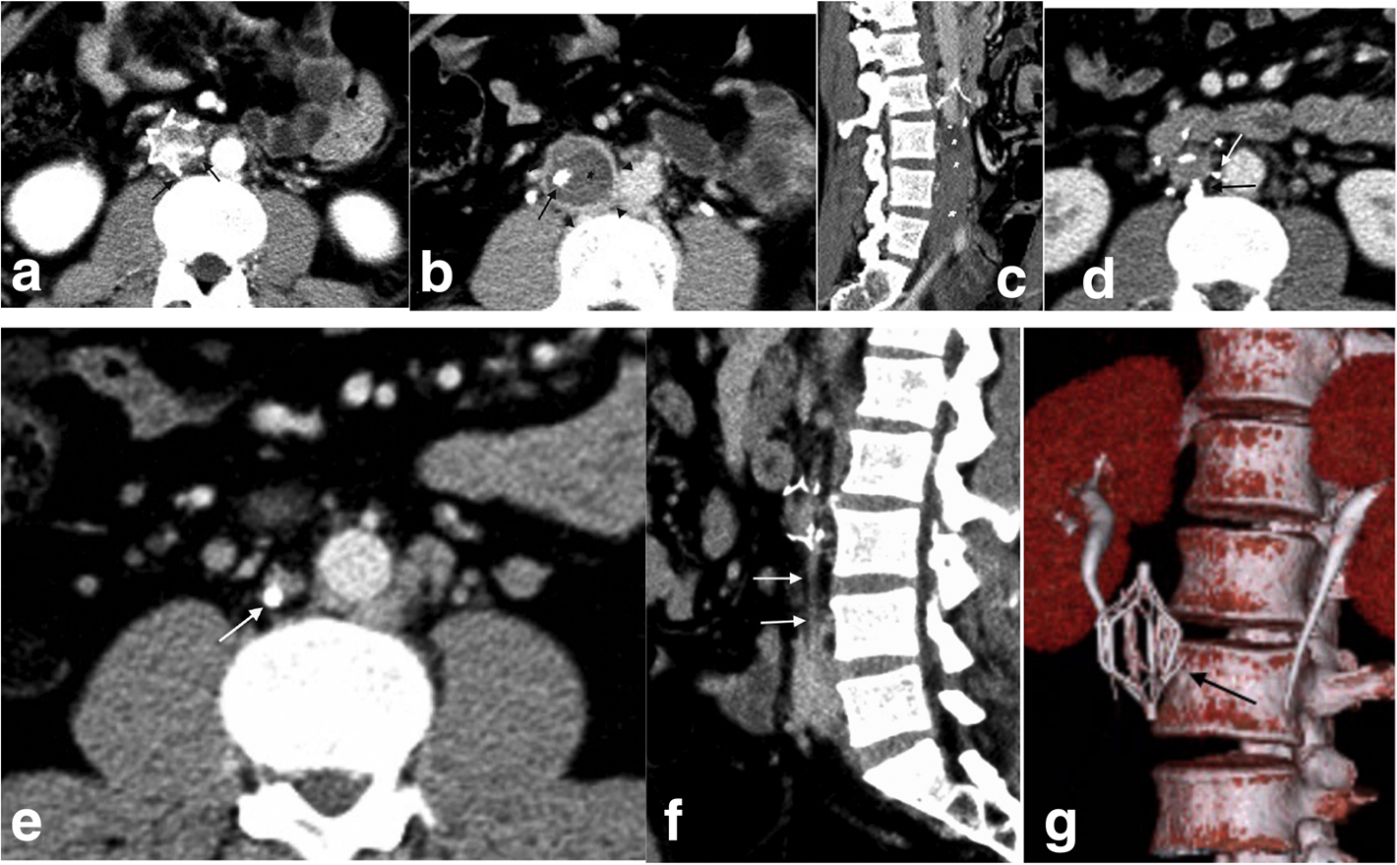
A case of traumatic intracranial bleeding from a left-sided iliofemoral thrombosis. a–c Follow-up CT of the IVC was obtained 10 days after filter placement. Note the extensive thrombosis (asterisks) in the IVC below the filter and the thickened IVC wall (black arrows) (b). The black arrow (b) marks the tip of the filter. d–g The last CT imaging of the IVC was performed outside the IVC shows one of the struts eroding the lumbar body, exhibiting grade 3 protrusion. The IVC has atrophied below the filter (e, f). Arrow in D marks the tip of the filter. Double white arrows in E mark the IVC as a strip below the filter. The reconstructed CT imaging (g) shows a fractured strut of the filter (black arrow)
